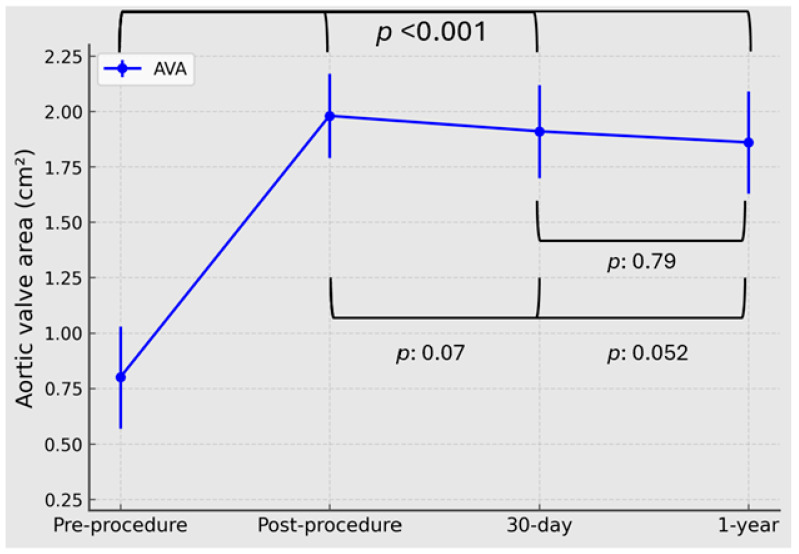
Serial aortic valve area after MyVal ViV-TAVI. Aortic valve area at baseline, post-procedure, 30 days, and 1 year after transfemoral MyVal valve-in-valve transcatheter aortic valve implantation. Values are shown as mean ± SD. Overall time effect: *p* < 0.001. Pairwise comparisons: post-procedure vs. 30 days, *p* = 0.07; post-procedure vs. 1 year, *p* = 0.052; 30 days vs. 1 year, *p* = 0.79. Observed echocardiographic sample sizes contributing data at each time point were n = 68 at baseline, n = 68 post-procedure, n = 64 at 30 days, and n = 59 at 1 year.
